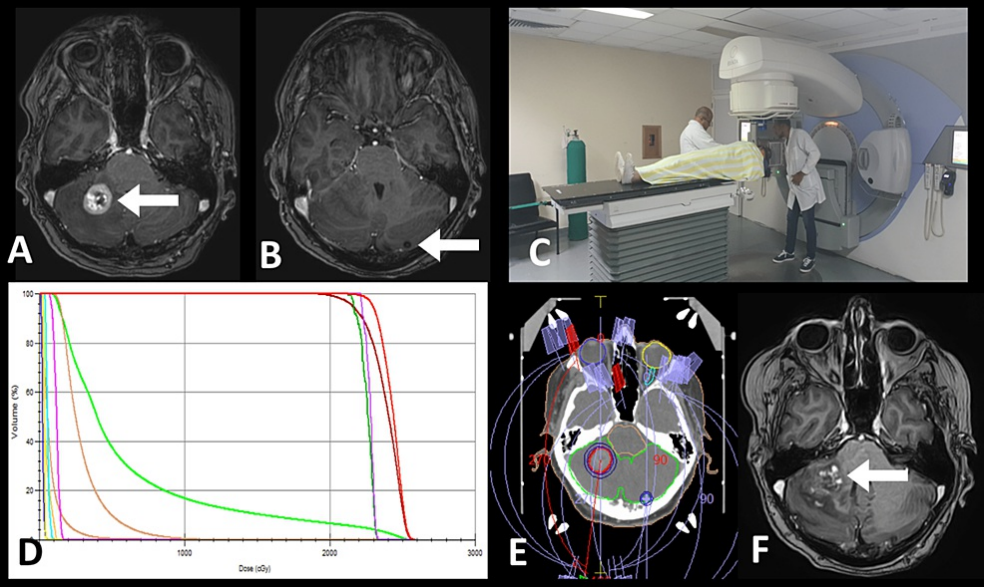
Patient with multiple breast cancer metastases. A: MRI planning where the largest lesion (arrow) is observed at the level of the right cerebellar hemisphere. B: The smallest hypo-intense lesion (arrow) is observed at the level of the right cerebellar hemisphere. C: Positioning of the patient in the linear accelerator (LINAC). The planning CT image is observed with the treatment arches. C: Positioning of the patient in the LINAC. D: Dose/volume histogram. E: Planning CT. F: MRI C+ at nine months of treatment where partial regression and necrotic center, as well as slight perilesional edema, are observed (arrow).
